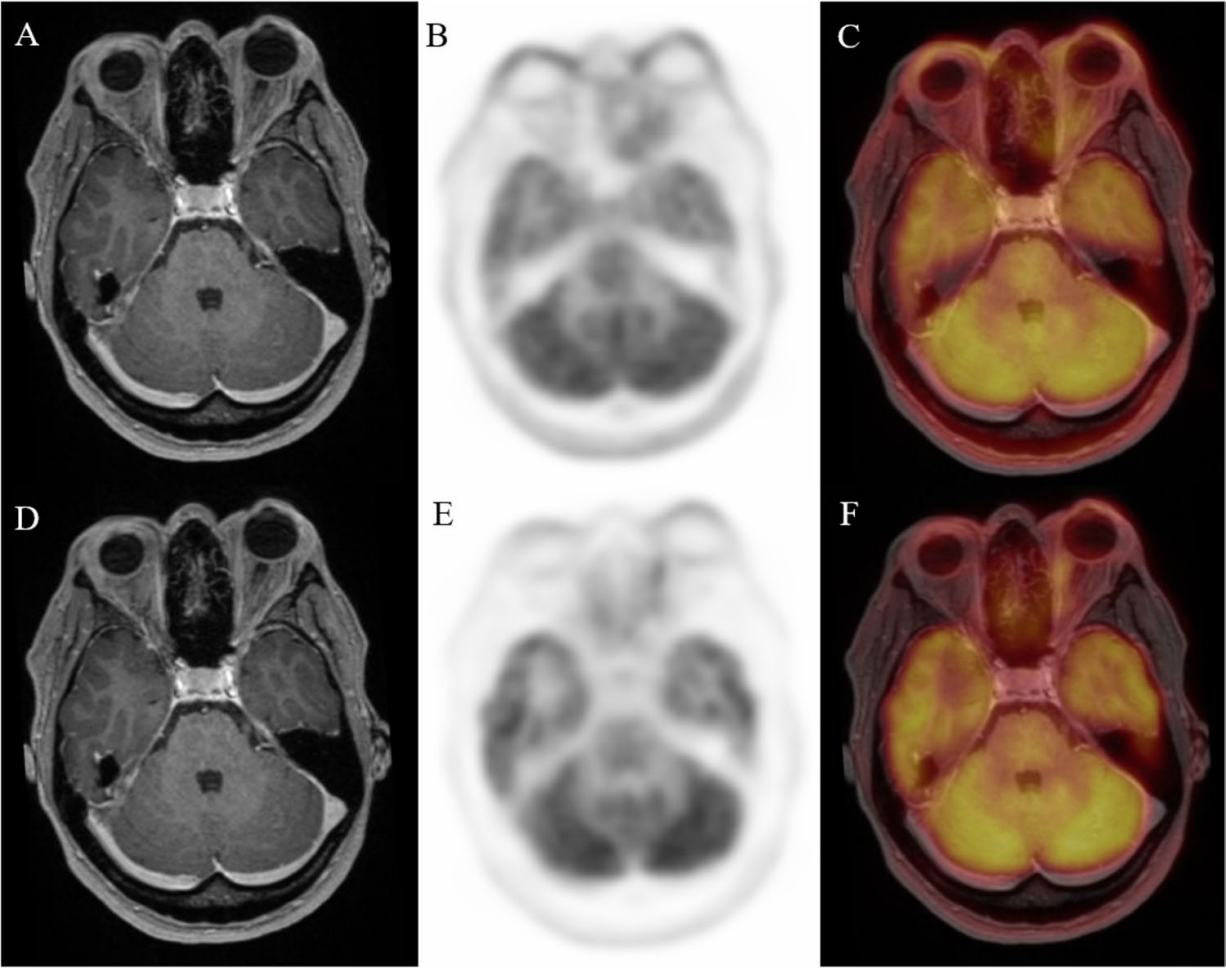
Transaxial MRI (panels A, D), PET (panels B, E), and fused slices of a 21-year-old patient with moderate, mainly left-sided GO reveal orbital tissue hypertrophy and ^18^F-FDG uptake, both more pronounced on the left side. The latter is more distinct on early static (panels A–C) vs. late static images (panels D–F)
